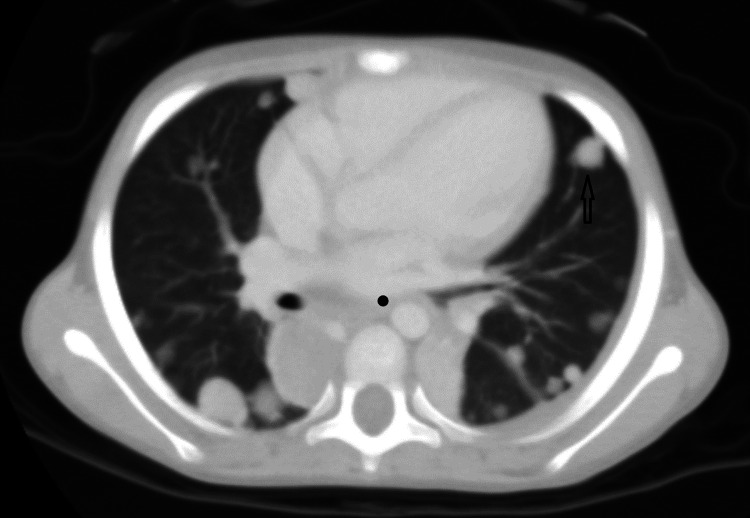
CT axial lung window (19-02-2024) showing multiple pulmonary metastatic deposits. CT, computed tomography.
